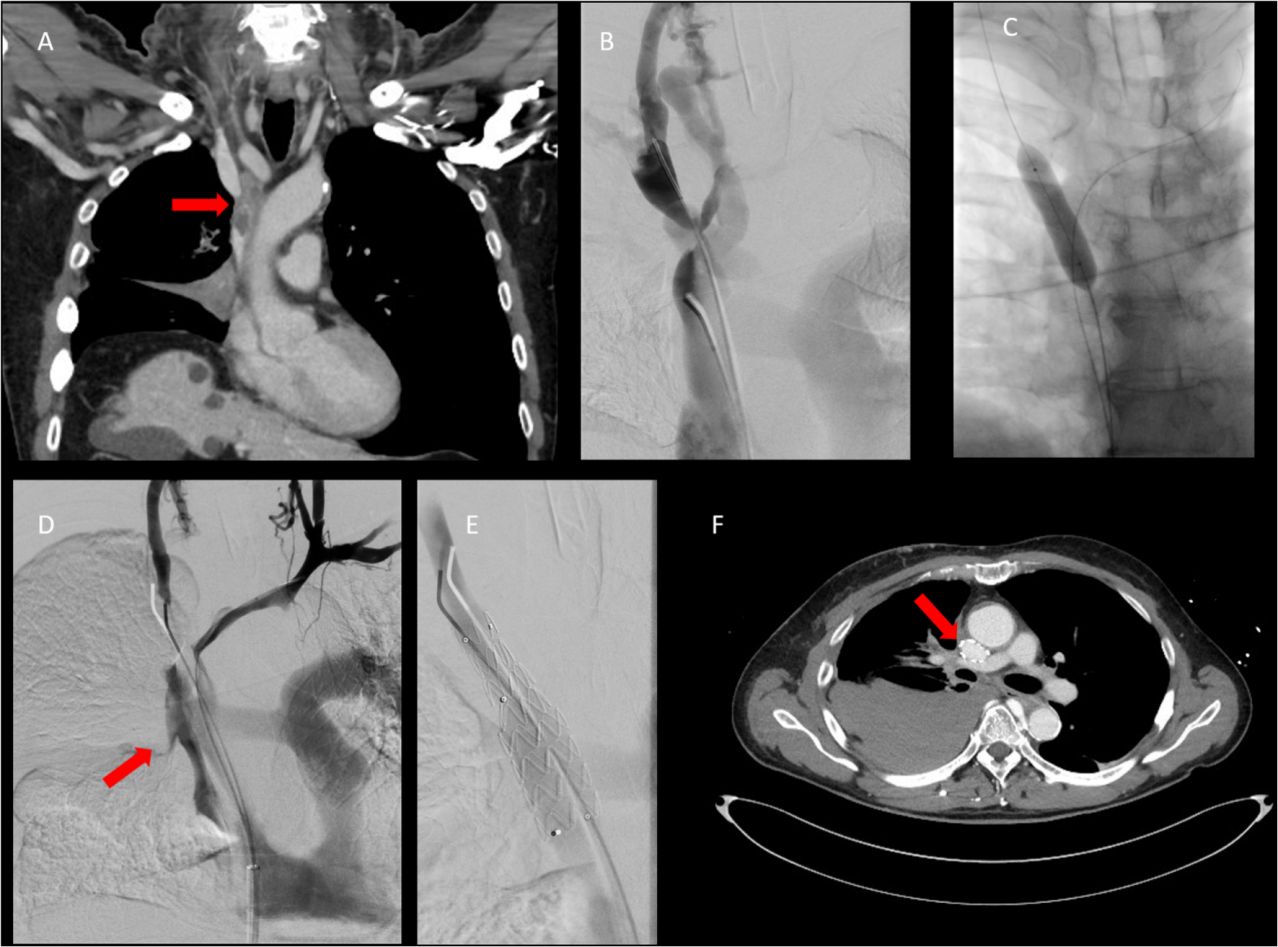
70-year-old male patient with bronchial adenocarcinoma and tumor-related high-grade stenosis of the superior vena cava with signs of SVC syndrome. A: Coronal contrast-enhanced CT showing near complete occlusion of the superior vena cava; B: Phlebography showing extensive venous bypass circulation; C: Ballooning of stenosis with a 12 mm balloon; D: Iatrogenic rupture of the superior vena cava (red arrow) with bleeding in the pleural cavity; E: Immediate implantation of a 20 mm endograft (Medtronic Endurant) at the rupture site with subsequent control; F: Axial slice of the control-CT; right-sided hemothorax with well-deployed endoprosthesis in the superior vena cava (red arrow)
